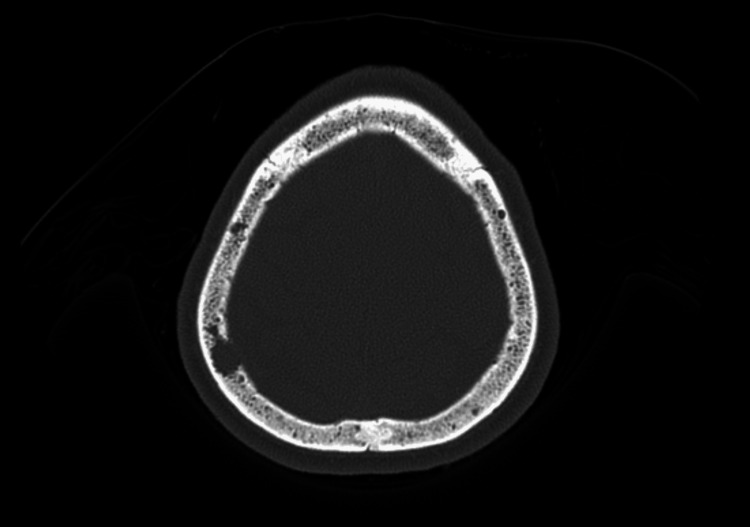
Cross-sectional view of CT skull with multiple lytic bony lesions
